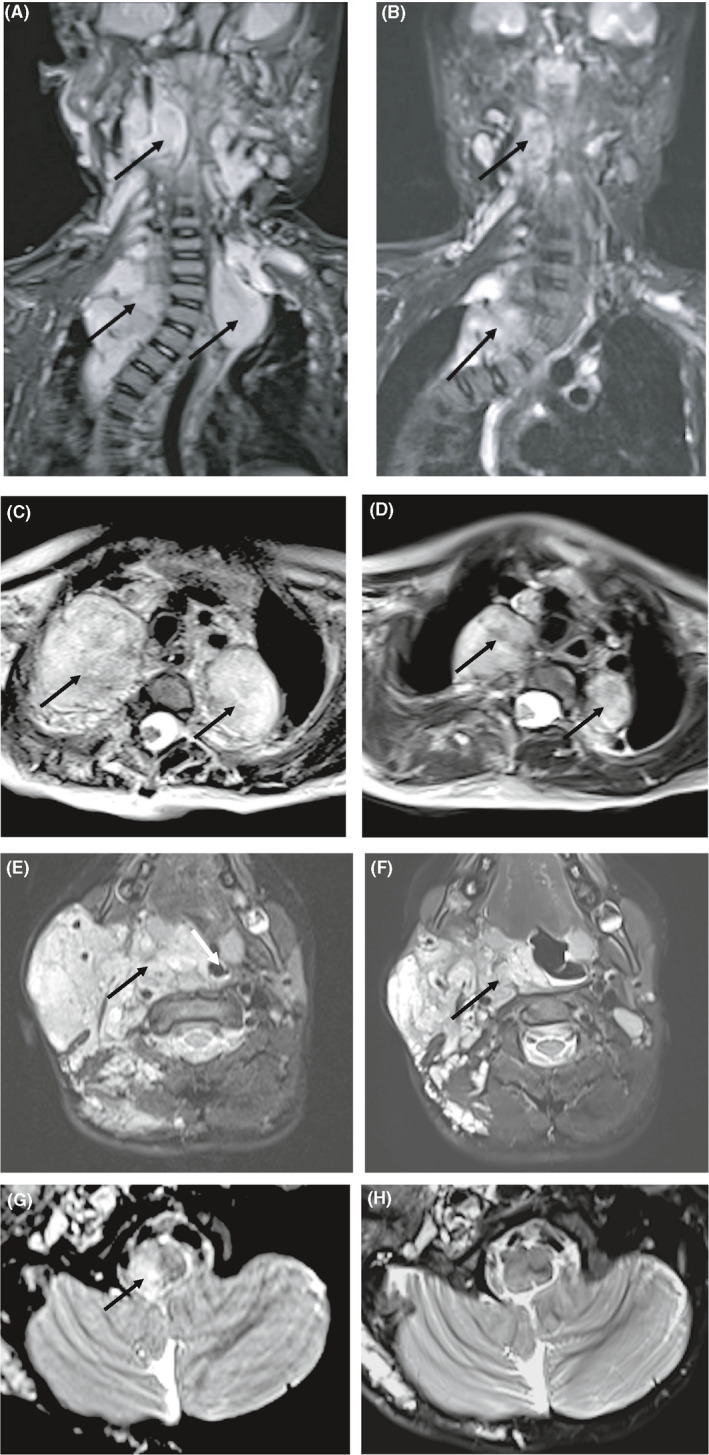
Radiographic Change During Trametinib Therapy. *Black arrow indicates lesion. (A) Case 2 – Coronal STIR image in a 21 month old female with progressive neurofibromas of neck and mediastinum prior to trametinib therapy. (B) Case 2‐ Radiographic improvement (volumetric partial response) after 26 months therapy with trametinib. (C) Case 2‐ Axial T2 image demonstrates the bilateral posterior mediastinal masses prior to trametinib. (D) Case 2‐ Radiographic improvement (volumetric partial response) after 26 months therapy with trametinib. (E) Case 1‐ Axial fat‐saturated T2 image in a 4 year old female with progressive neurofibromas of the face prior to trametinib. There is significant displacement and narrowing of the nasopharyngeal airway. White arrow indicates the nasopharyngeal airway. (F) Case 1‐ Radiographic improvement (volumetric partial response) after 17 months therapy with trametinib. A laryngeal mask airway was used for this sedated MRI and distends the displaced nasopharyngeal airway. (G) Case 1‐ Axial fat‐saturated T2 image demonstrates a low grade glioma of the right posterior medulla prior to trametinib therapy. (H) Case 1‐Improvement of size and signal of the low grade glioma after 17 months therapy with trametinib (RAPNO partial response)
